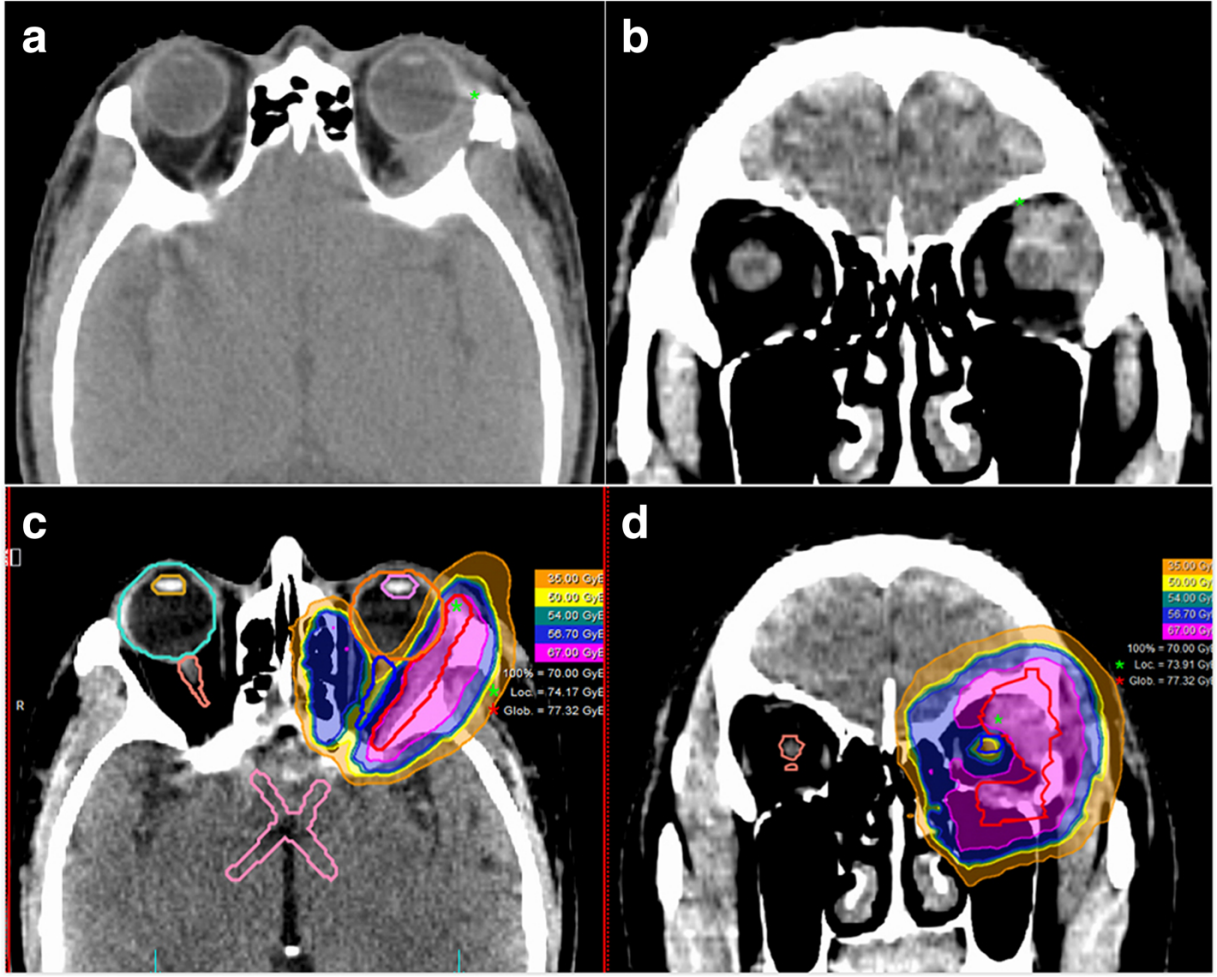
Axial (a) and coronal (b) views of a post eye sparing surgery CT scan of a patient with left lacrimal gland ACC. Axial (c) and coronal (d) views of a typical intensity-modulated carbon ion radiotherapy treatment plan
